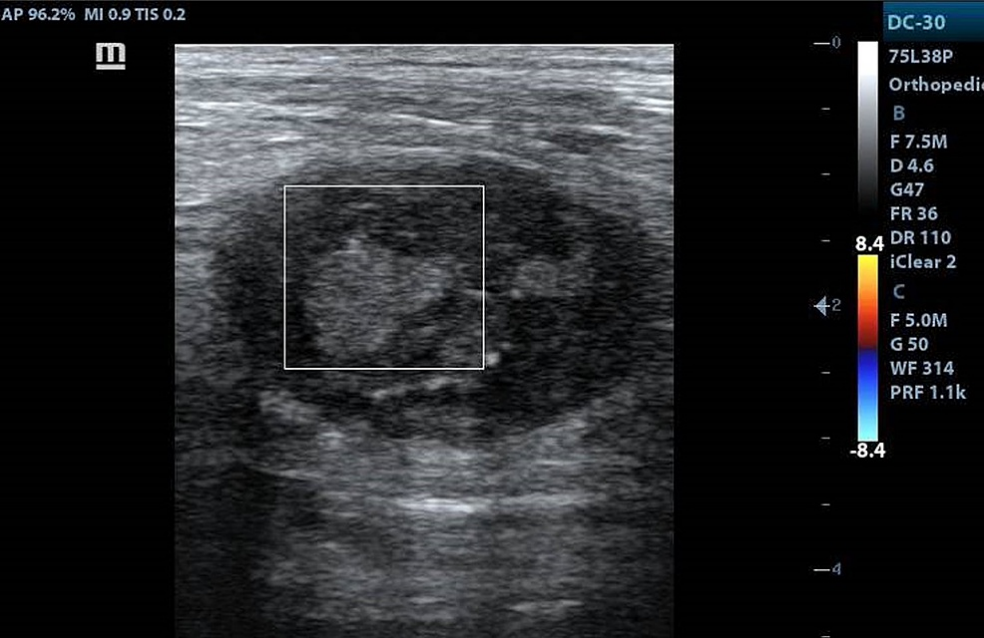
High-resolution ultrasonography image at one month follow-up demonstrating ill-defined hyperechoic areas within the lesion favored to represent spontaneous intralesional hemorrhage.
